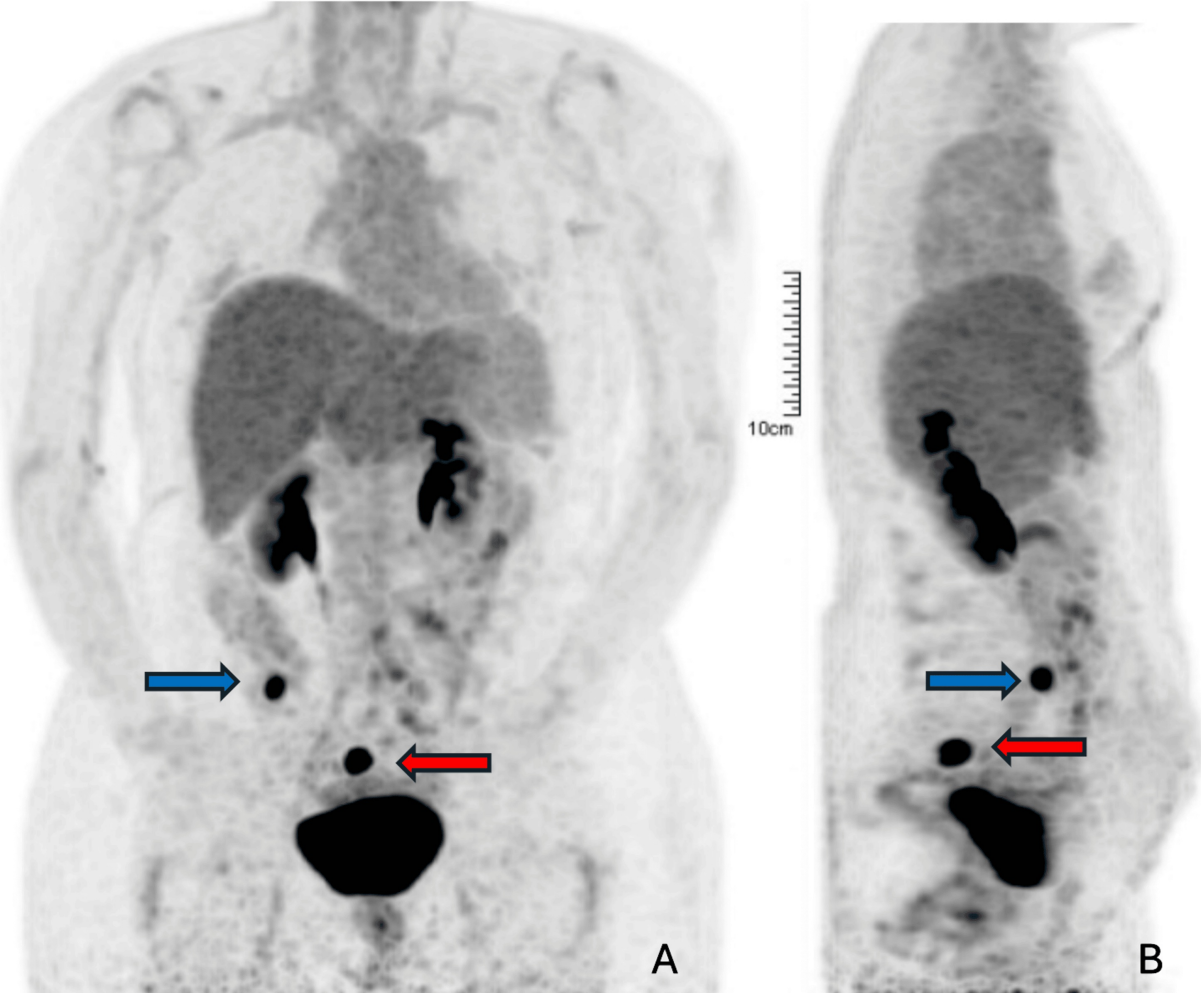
PET-scan showing the first radiologic evidence of disease recurrence, detected six months after neurological symptoms began (August 2025) (A) Coronal FDG-PET image showing a focal hypermetabolic area in the right iliac fossa (blue arrow; SUVmax 18.27) and a second hypermetabolic focus in the presacral region (red arrow; SUVmax 19.39), both adjacent to intestinal loops. (B) Sagittal FDG-PET view confirming the same hypermetabolic foci (blue and red arrows), persistent and with increased uptake on delayed imaging, findings suspicious for metastatic peritoneal implants. PET: positron emission tomography; FDG: fluorodeoxyglucose; SUVmax: maximum standardized uptake value
